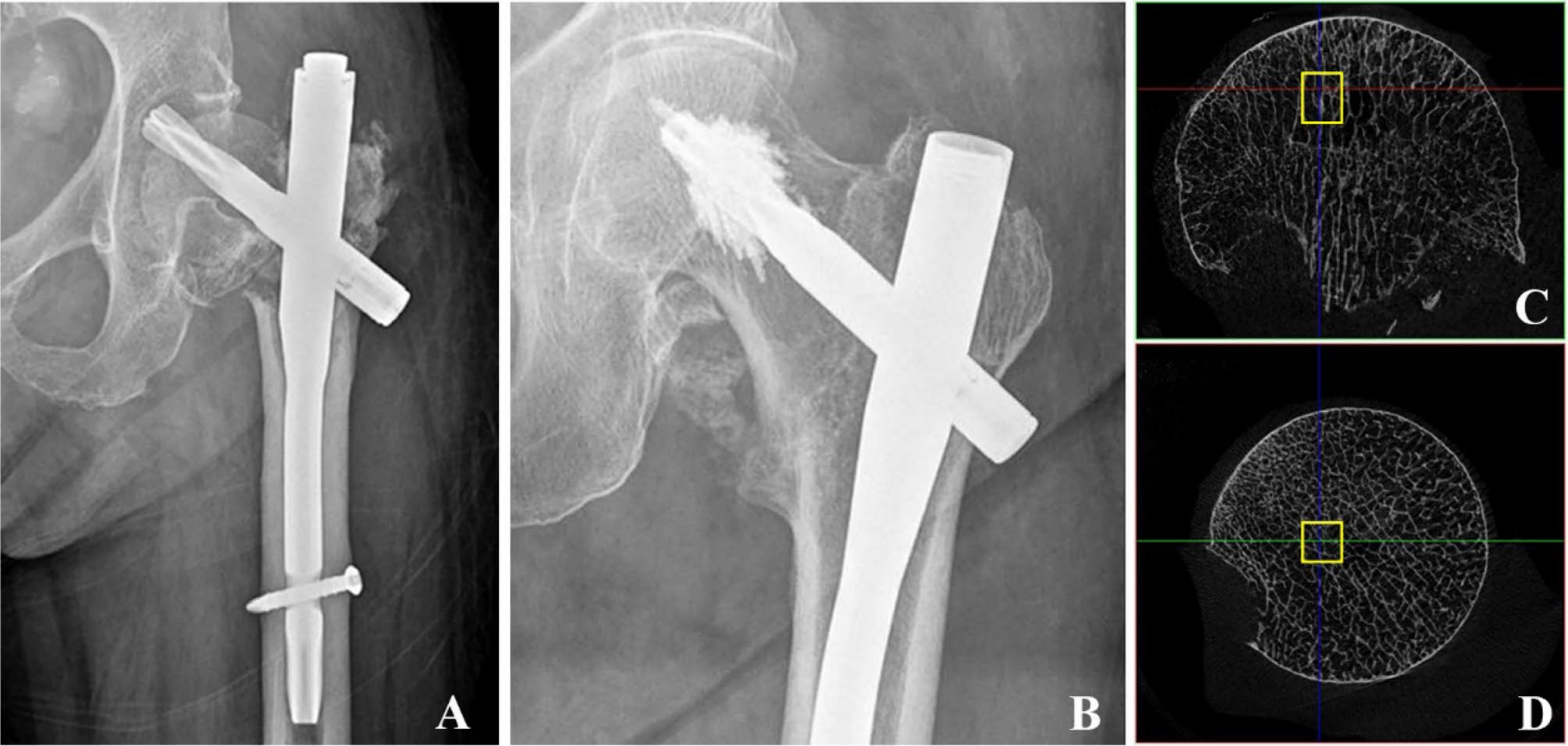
(A) Radiographic image of a blade cut-out extending from the femoral head into the acetabulum^29^. (B) Radiographic image of a completely recovered intertrochanteric fracture after cement-augmented PFNA fixation^8^. Figures were reproduced with permission from Elsevier. (C) and (D) VOI of trabecular bone segmented from the femoral head. The axis of VOI is aligned with the main compressive trabecular direction.
